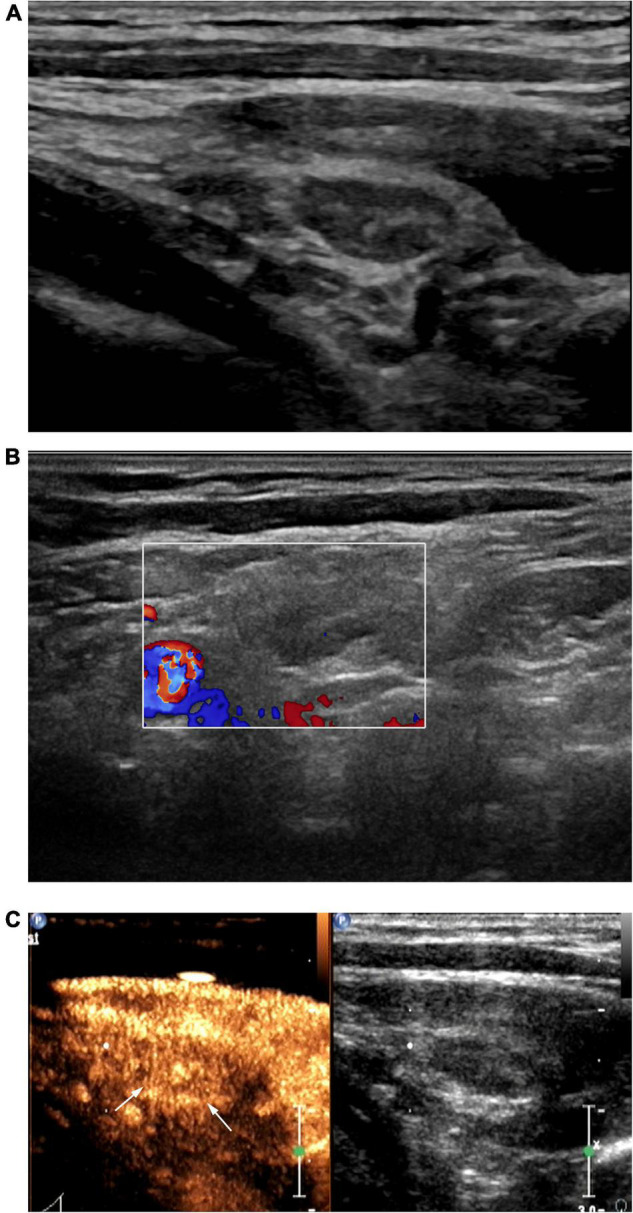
Patient with left cervical tuberculous lymphadenitis (CTL) (30-year-old man). Conventional ultrasound (US) showed type I cervical tuberculous lymphadenitis (CTL), which remains classified as type I after contrast-enhanced ultrasound (CEUS). US-guided fine-needle aspiration was performed to obtain a small amount bloody fluid. Pathological analysis of this was consistent with the manifestations of type I CTL. (A) Two-dimensional US indicated a lymph node 1.1 × 0.5 cm in size with a clear border, thickened cortex, uniform internal echoes, visible hilum of lymph node. (B) Punctate blood flow signals were observed in the center of the lymph node. (C) Under peak CEUS enhancement, homogeneous enhancement of the lymph node was observed.
